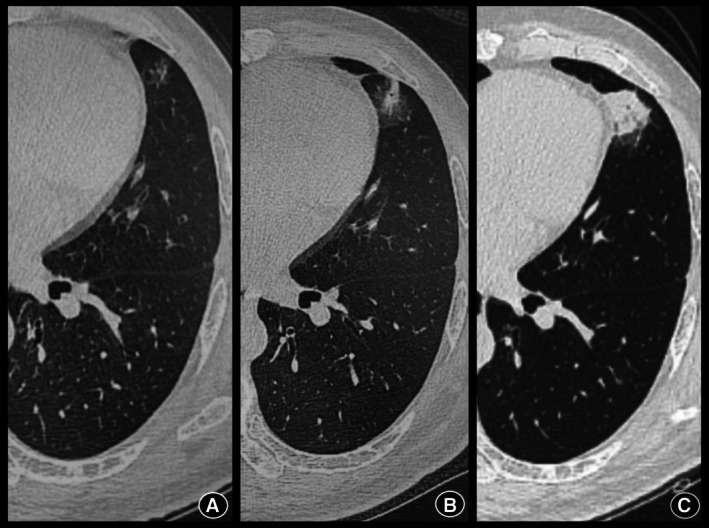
Serial chest CT images of the left upper lobe lesion. (A) September 2020: Focal ground‐glass opacity in the lingular segment. (B) February 2022: Interval enlargement to 25 mm × 21 mm with heterogeneous density. (C) June 2023: 29 mm × 26 mm mixed‐density nodule abutting the pericardium, with heterogeneous enhancement.
